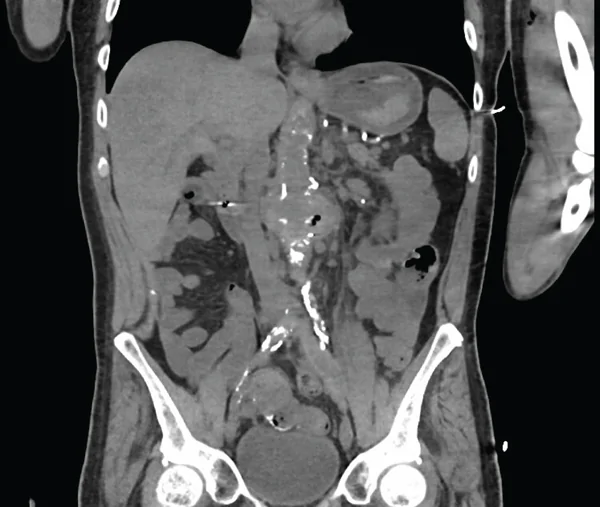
Repeat CTA of the abdomen and pelvis showed increased induration around the proximal graft along the mid‐aorta, with new findings of perigraft and a small area of contrast pooling anterior to the top of the graft.
